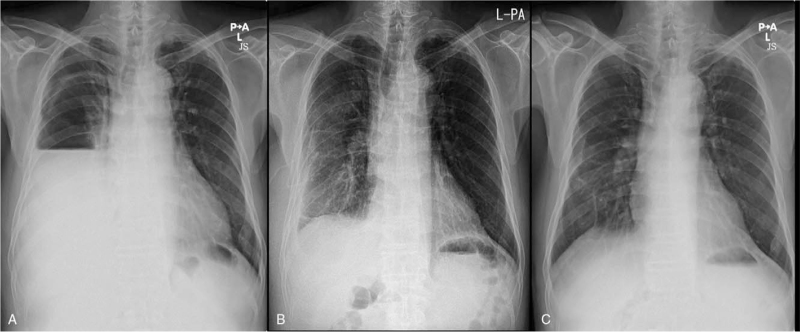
Chest X-ray findings. (A) Massive pleural effusion and a collapsed lung in the right lower lung field on admission. (B) Improved pleural effusion after thoracic drainage on the 11th day of admission. (C) Resolution of the empyema after 1 year.
